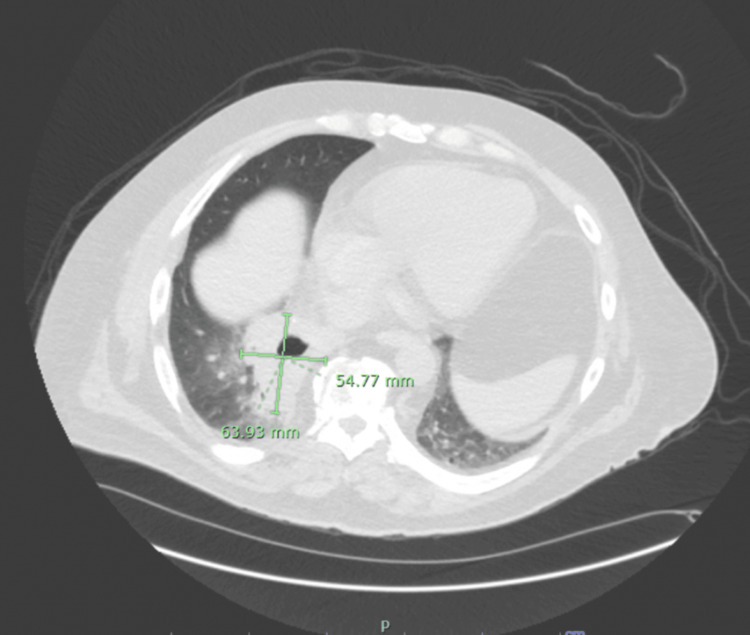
CT scan of the lung on day 1 showing a thick-walled cavitated lesion measuring 54.77 mm x 63.93 mm. Image obtained at our institution, Baystate Medical Center, and used with family consent.
